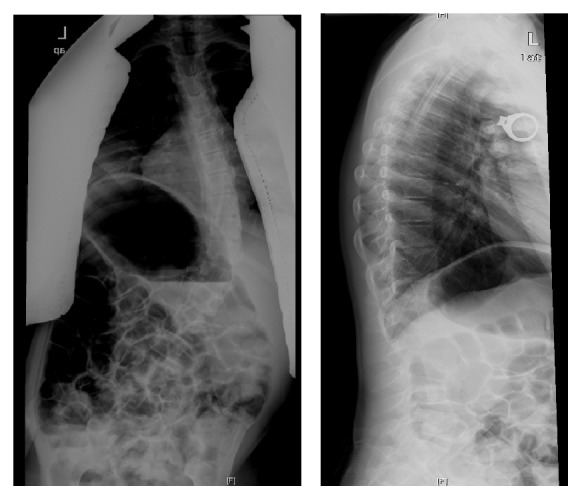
Preoperative radiographs showing a 54-degree scoliosis deformity in a patient with Rett syndrome. Patient has coronal imbalance. The pelvis is level.
